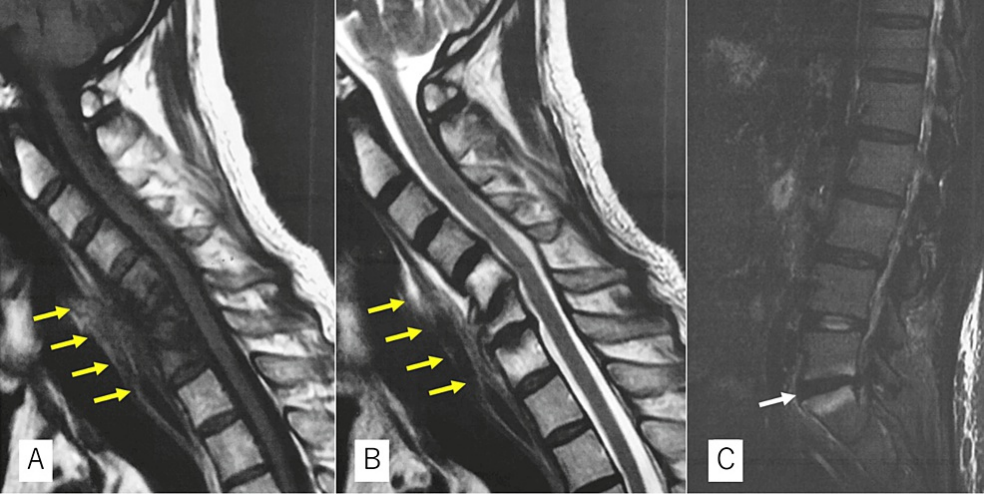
Preoperative MRI scan Magnetic resonance image showing low signal intensity with T1-weighted images (A) and high signal intensity with T2-weighted images (B) from C5 to C7 with retropharyngeal swelling (yellow arrows). The L5-S1 segment also showed a high signal intensity change in the short tau inversion recovery (STIR) image without disc involvement (white arrow) (C).
